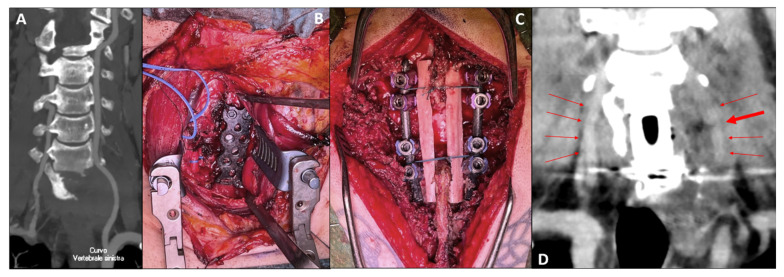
Vascular assessment and intraoperative reconstruction. (A) Preoperative CT Angiography. Coronal reconstruction demonstrating the critical relationship between the tumor and the left vertebral artery (VA). The vessel appears significantly displaced by the tumor mass. (B) Intraoperative view (Anterior Stage). Final construct showing the CFR-PEEK vertebral body replacement cage (KONG® VBR) and anterior cervical plate (BlackArmor®). (C) Intraoperative view (Posterior Stage). Final posterior stabilization. The image displays the CFR-PEEK pedicle screws and rods (VADER® system) in position. A double structural fibular allograft is visible, securely tethered to the rods to promote posterior fusion. (D) Postoperative CT Angiography. Coronal reconstruction confirming the continuity of both vertebral arteries (red arrows). While the right VA shows normal caliber and enhancement, the repaired left VA remains patent but exhibits reduced contrast density, indicating lower flow velocity. A focal stenosis is clearly identifiable at the anastomotic site (large red arrow), consistent with the vascular repair described.
